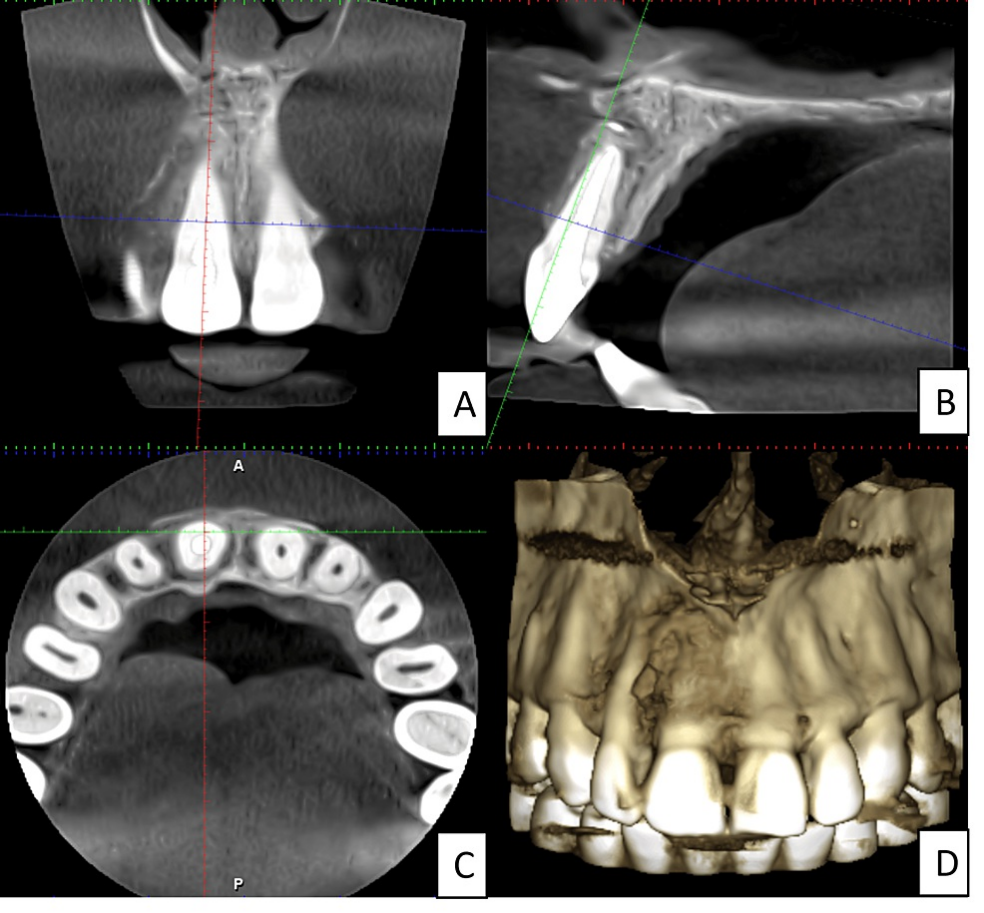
(A) One-year follow-up CBCT showing the coronal section with tooth number 11, (B) CBCT showing the sagittal section with tooth number 11, (C) CBCT showing the axial section with tooth number 11, (D) CBCT showing the skeletal view CBCT: Cone-beam computed tomography
